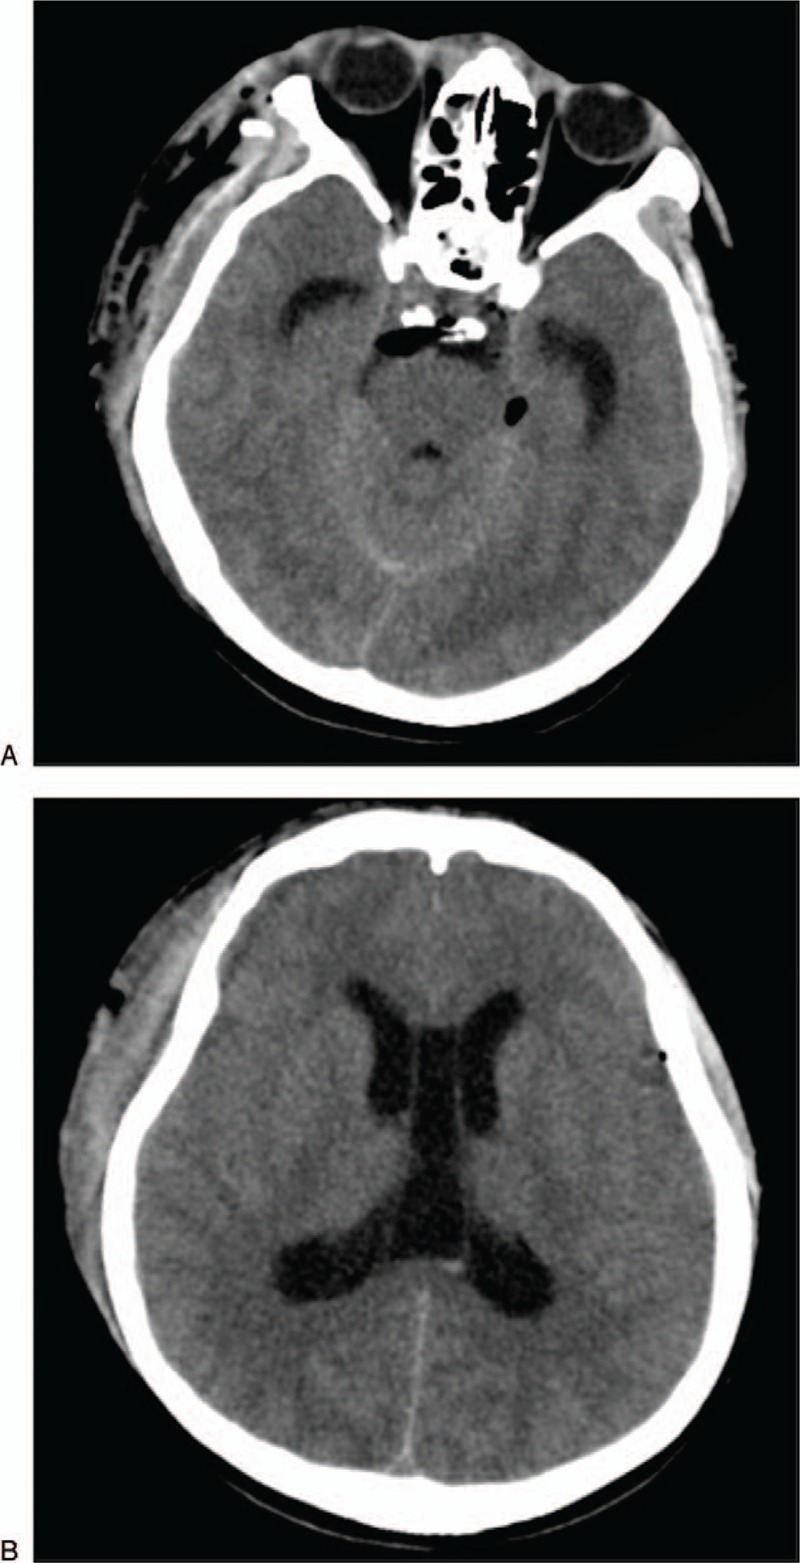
(A and B) Computed tomography performed 3 days later shows perimesencephalic pneumocephalus and evident enlargement of the ventricular system. The bilateral cistern ambiens vanish.
